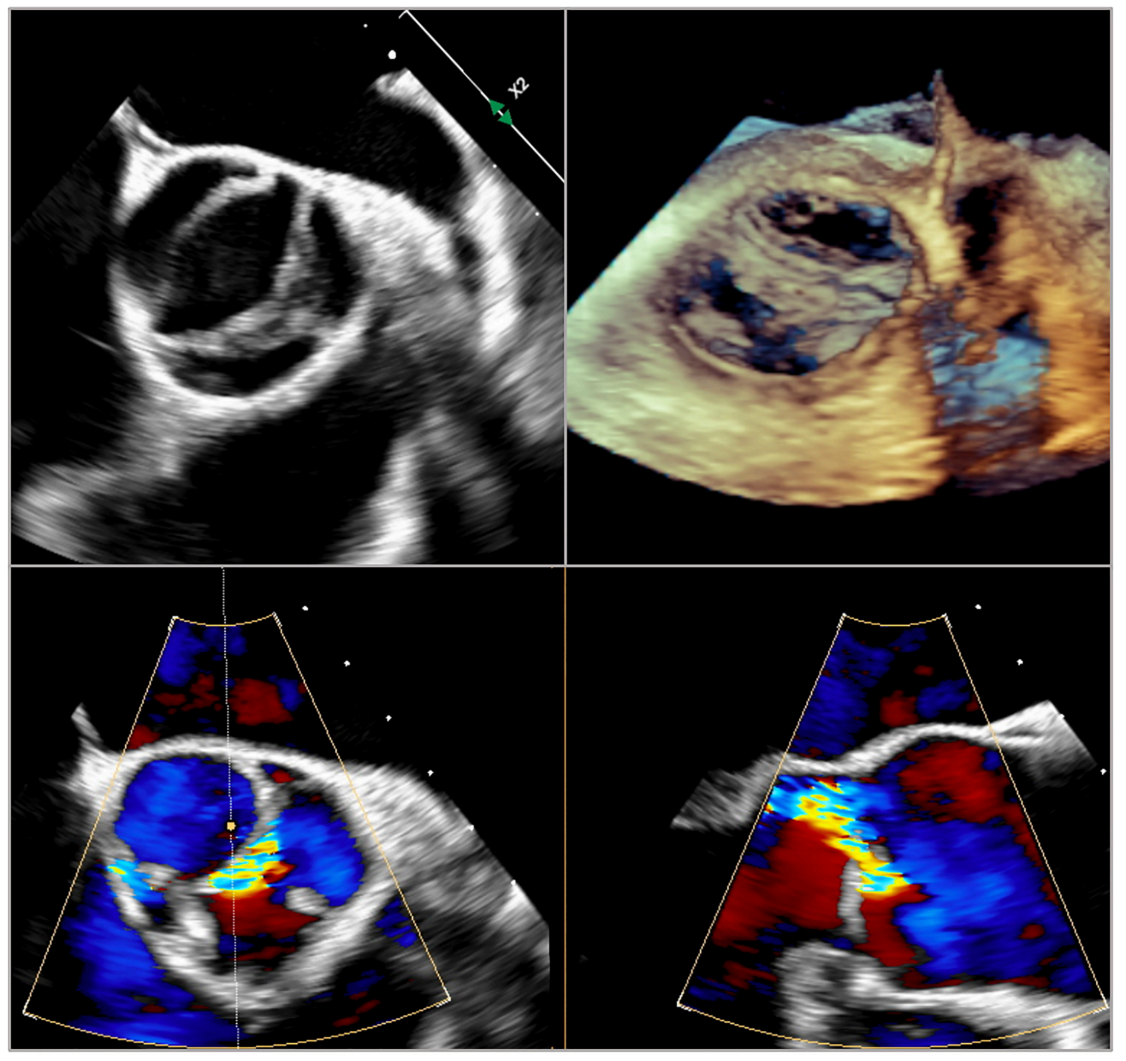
Transesophageal echocardiographic images of bicuspid aortic
